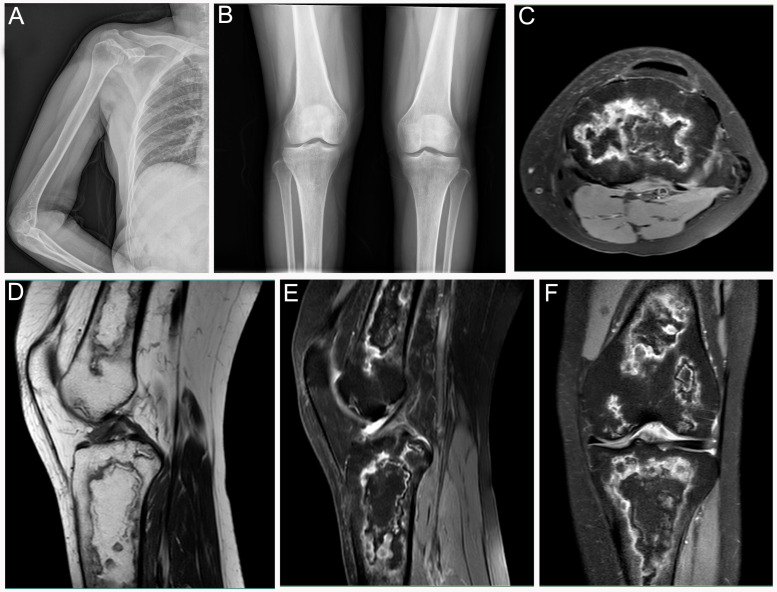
Patient 3: (A,B) X-ray films of the right upper extremity and knee joints. (C–F) Sagittal T1-weighted image of left knee showed irregular low-SI areas with low-SI margins. Edema zone surrounding infarcted bone of knee joint presented high SI on transverse, sagittal, and coronal T2-weighted fat-suppressed images.
